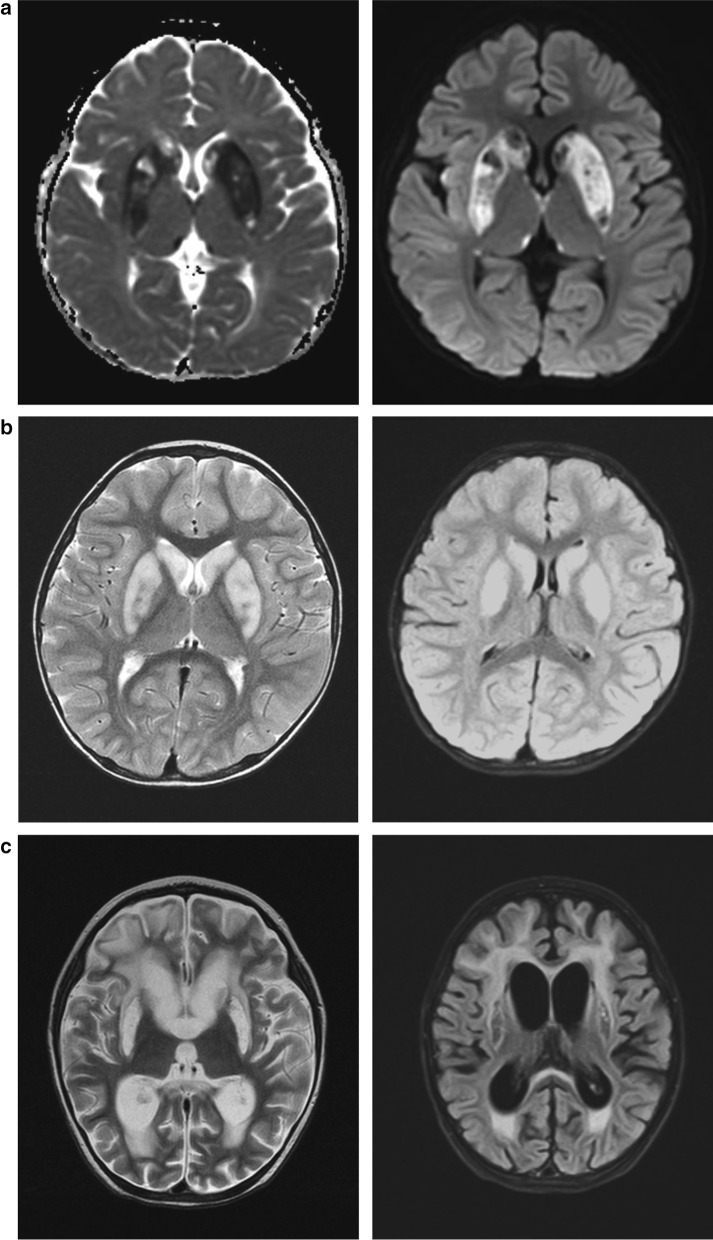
Brain magnetic resonance imaging (MRI) of the three patients. a Brain MRI of Patient 1 showing abnormal signals in the bilateral basal ganglia, thalamus margin, hippocampus, midline frontal lobe, and temporal cortex. b Brain MRI of Patient 2 displaying abnormalities in the bilateral basal ganglia, thalamus margin, and brainstem. c Brain MRI of Patient 3 showing signal changes in basal ganglia and thalamus edge
